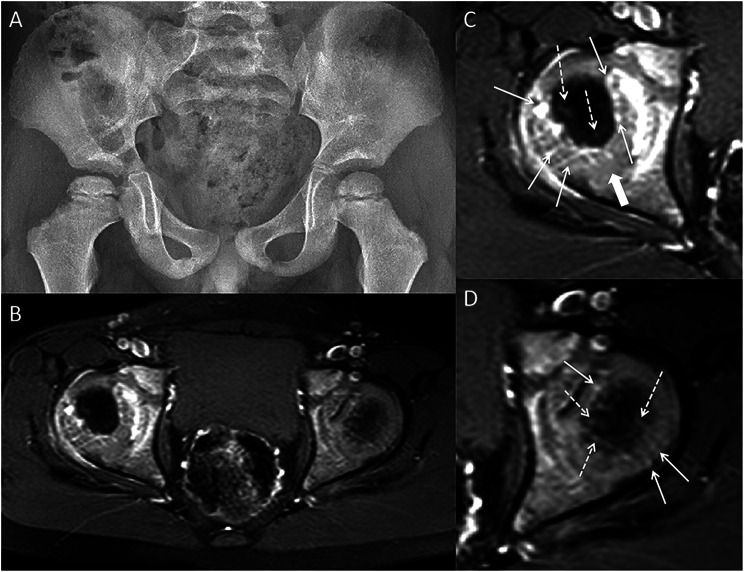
A 4-year-old boy with right LCPD. (A) The pelvic radiograph. (B) Axial enhanced MR image. (C-D) The enlarged images of the right and left FH. The ossification center vessels (dotted arrow) are significantly fewer than on the left side. However, the cartilaginous vessels (arrow) in the medial, lateral and posterior parts of the right FH exhibit a more increased grade and are thicker than those on the opposite side. Additionally, the distribution and course of some cartilaginous vessels are similar to those of the normal FH. A zone of relatively sparse vascularity exists in the posterior part (thick arrow). The cartilaginous vessels of the anterior part are the least in the four parts of the bilateral FH. MR = magnetic resonance, FH = femoral head, LCPD = Legg–Calvé–Perthes disease
